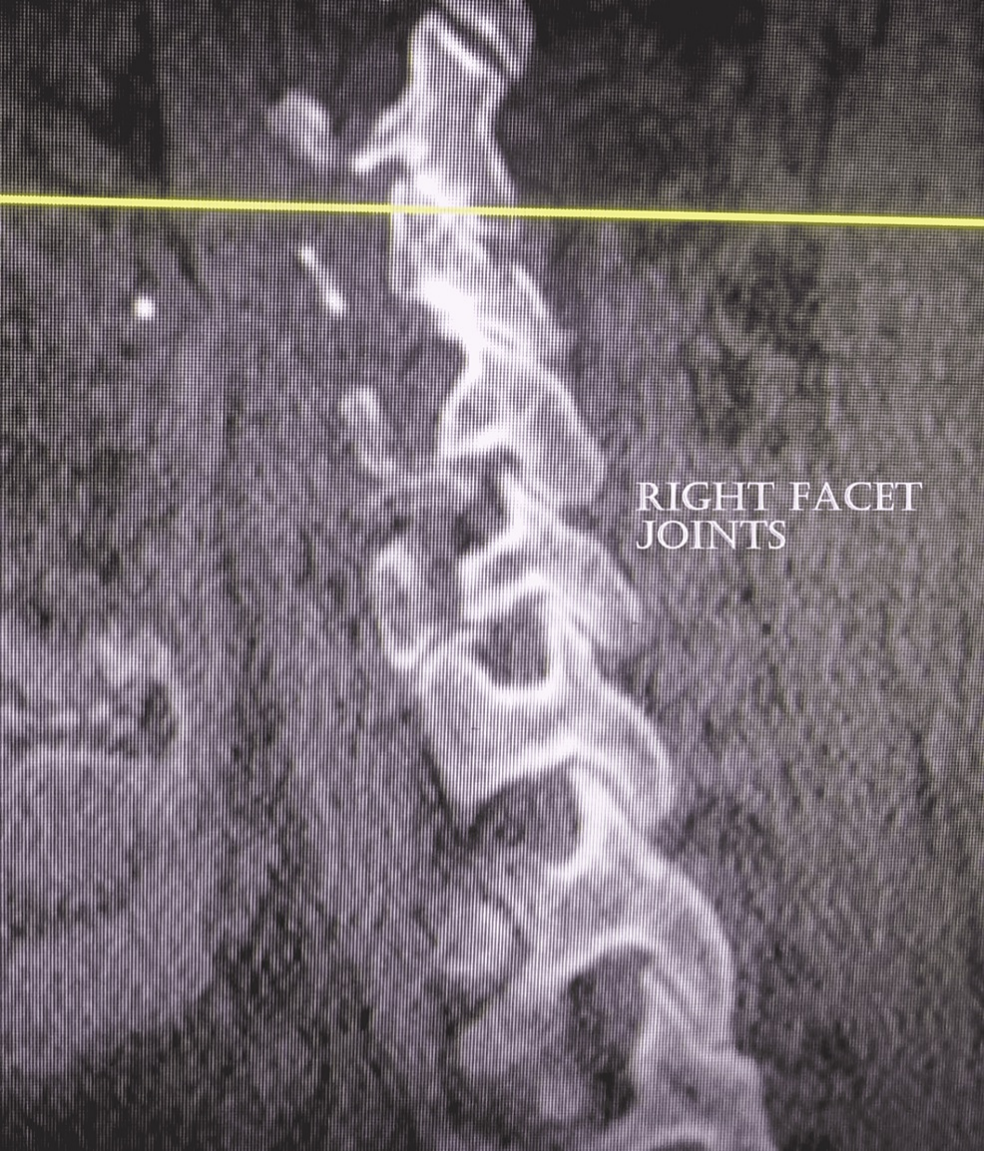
Sagittal CT image of the cervical spine showing right facet joints. Grade 2: joint space reduction, osteophytes formation, and hypertrophy.
